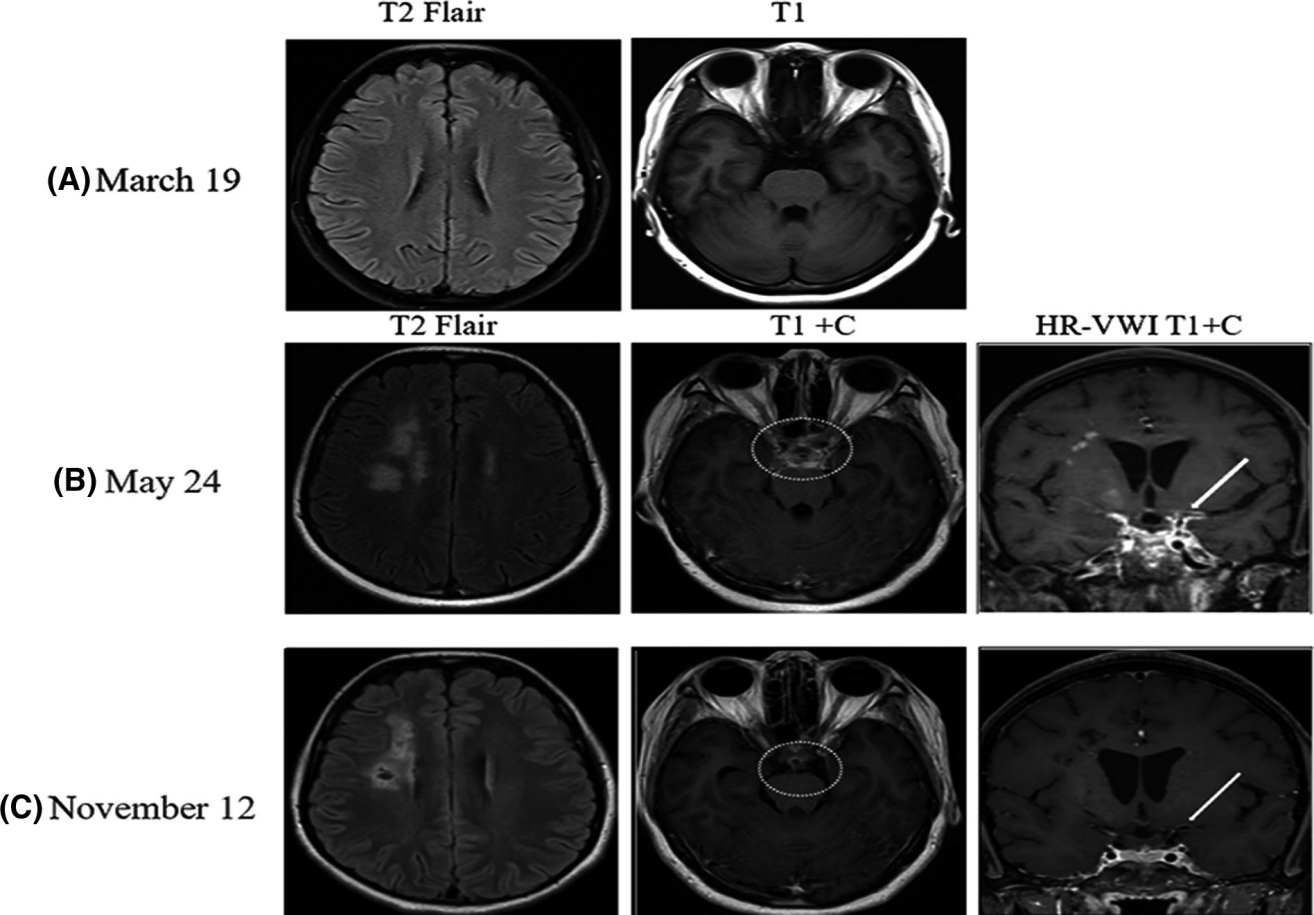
Brain imaging of the patient. (A) MRI before the onset showed normal. (B) MRI on the 24th day of the onset without effective antibiotic therapy showed the acute cerebral infarctions in the right basal ganglia and frontal lobe and meningeal enhancement in slope, saddle cistern, and interfoot cistern. (C) Follow‐up MRI showed marked improvement after the effective anti‐infective treatment (T1+Contrast showed the significantly improved meningeal enhancement, and HR‐VWI T1+C showed the enhancement of vascular wall after improved meningeal enhancement)
